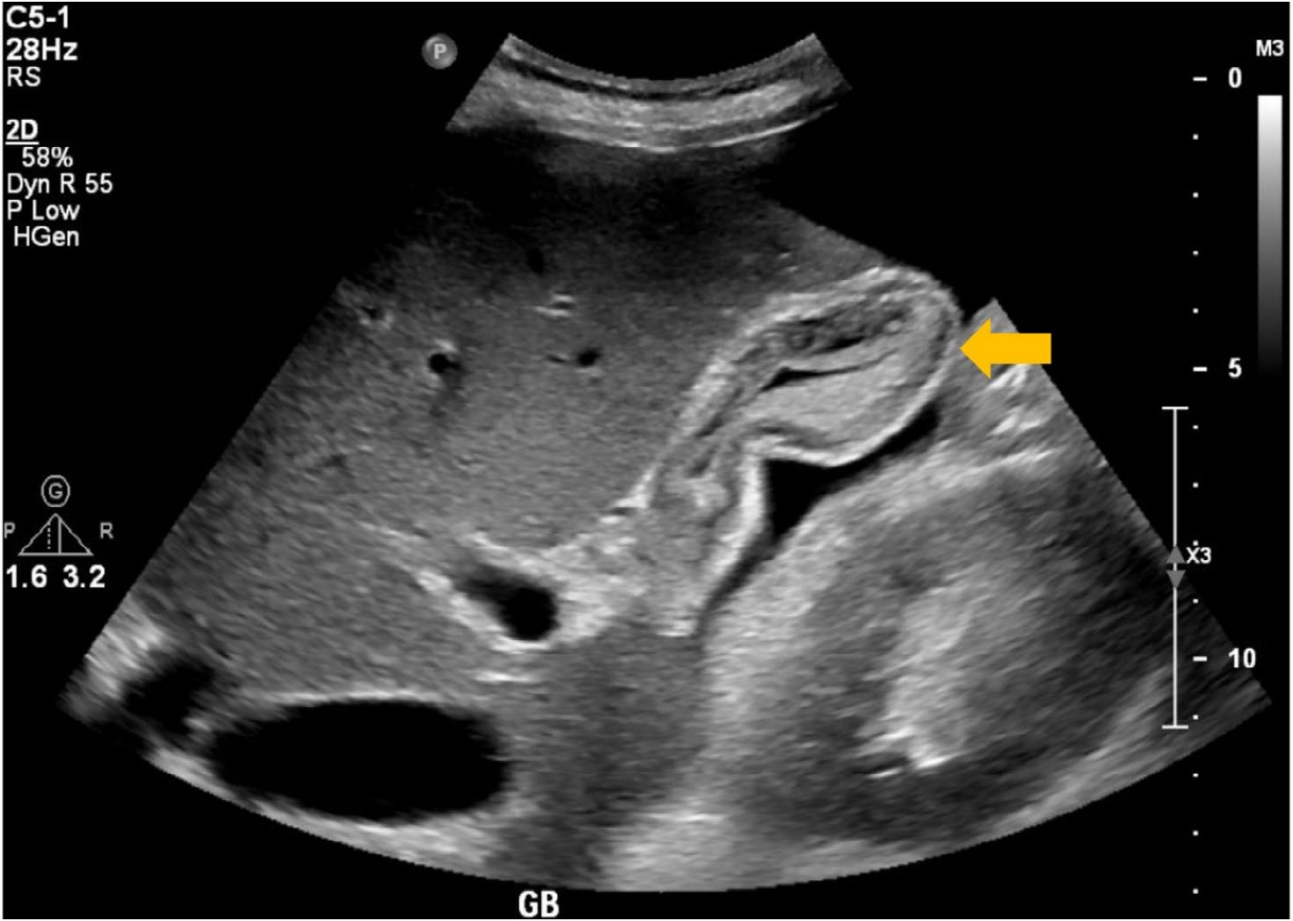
An ultrasound of the abdomen showing a thick and swollen gallbladder wall.
